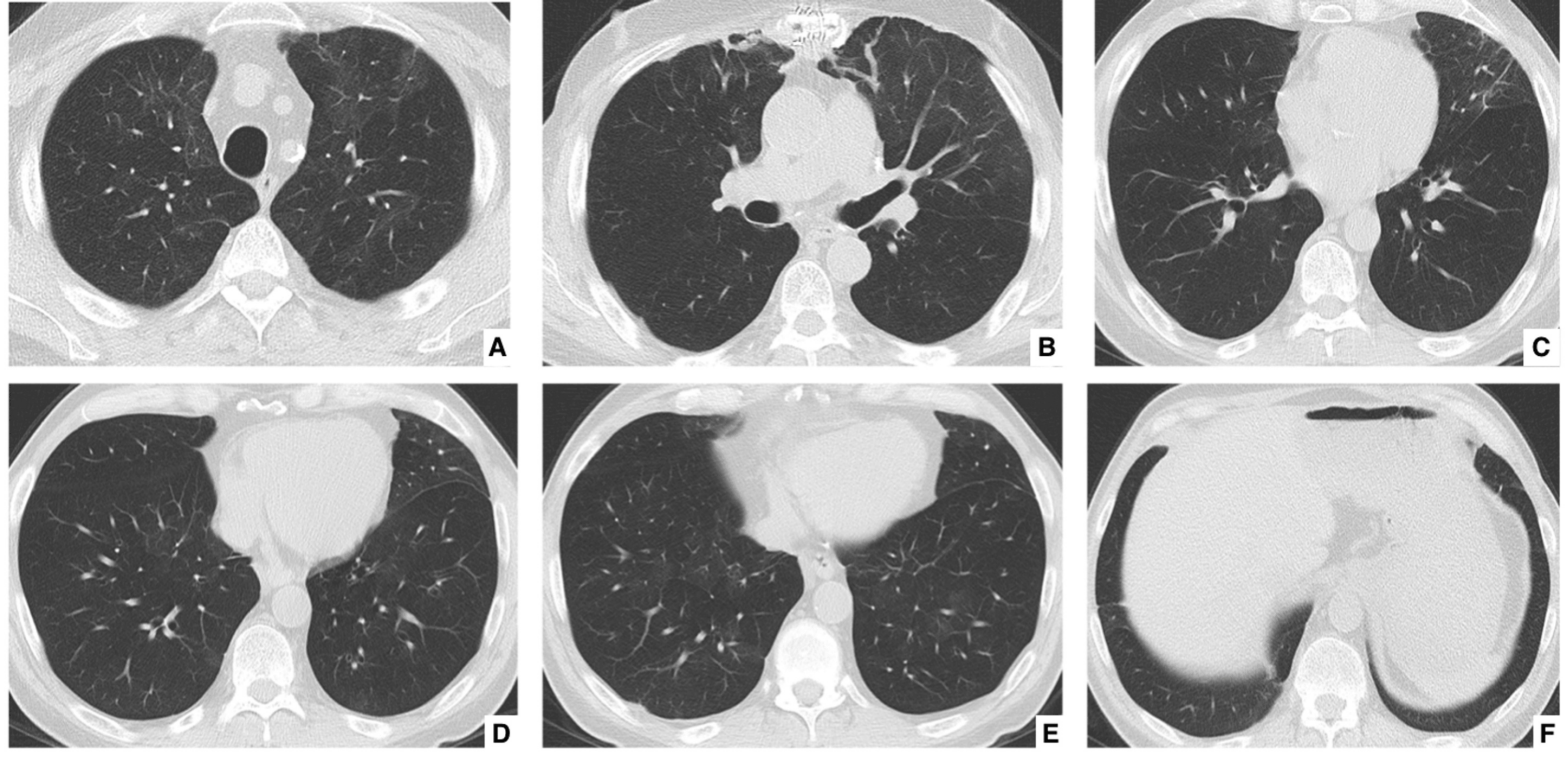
Post-everolimus discontinuation follow-up Follow-up axial chest CT images at the same anatomical levels (A–F) demonstrating resolution of the previously seen ground-glass opacities and consolidations.
